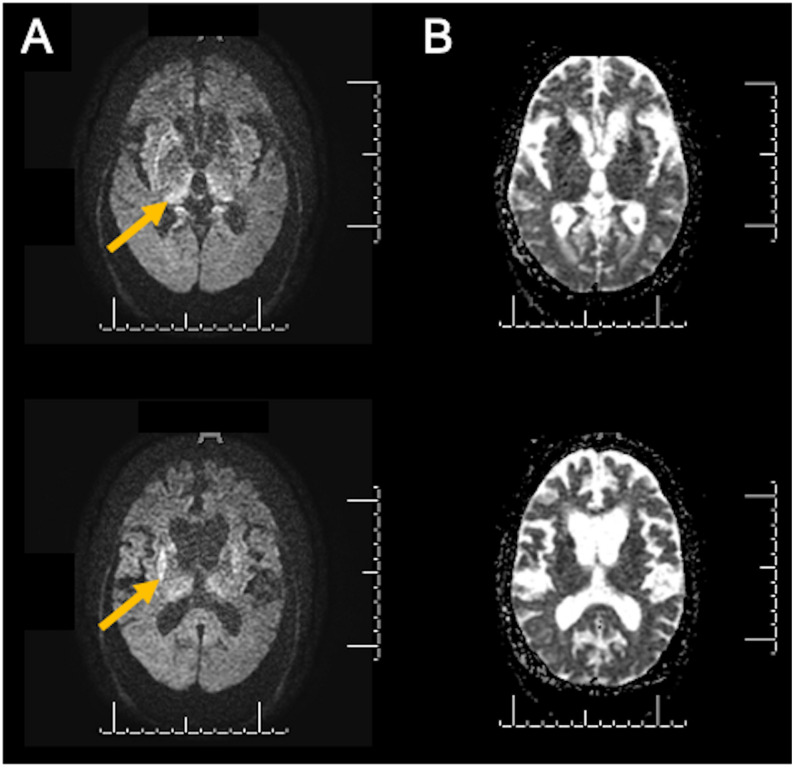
Diffusion-weighted images (DWI) from ABT (case 1) revealing enlarged ventricles and symmetric hyperintensities in the basal ganglia (A, yellow arrows). (B) shows corresponding apparent diffusion coefficient (ADC) hypointensities in the same territory.
